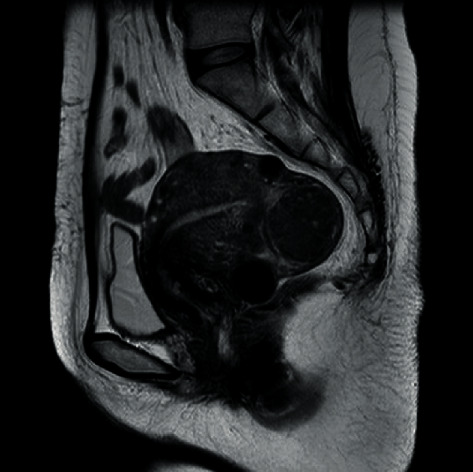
Magnetic resonance imaging was performed before uterine artery embolization. MR images show clearly visible fibroids and not tumors.
